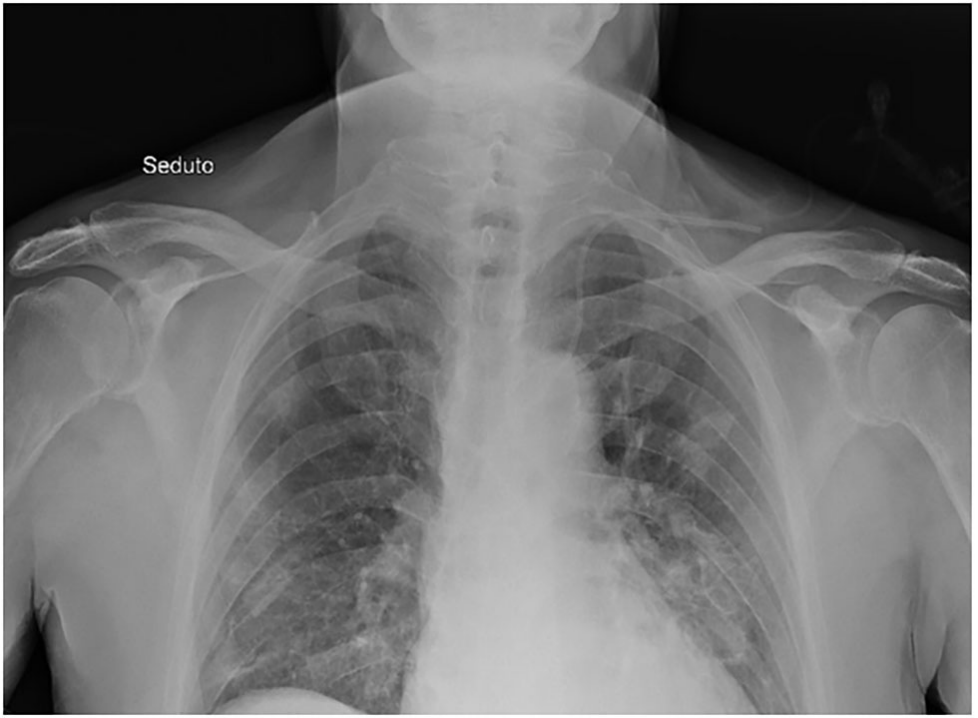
Chest X-ray. Chest X-ray description: small peripheral hazy opacity in medium field and more evident opacities at the medium-lower fields of both lungs, especially on the left side.
